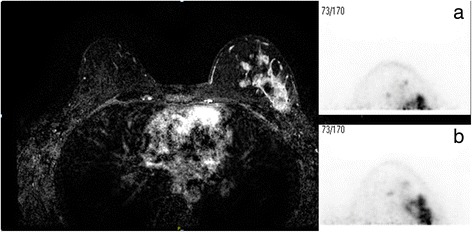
Contrast-enhanced MRI and db-PEM images of a patient with a large multi-focal breast cancer with greatest extend measured at 6.8 cm. The db-PET images acquired with the standard aperture (a) only show the anterior portion of the lesion, with an extent of 4.4 cm. With the large 220-mm aperture (b), there is more complete visualization of tumor extent (maximum extent of 6.1 cm) that closely matches that seen on the MRI
